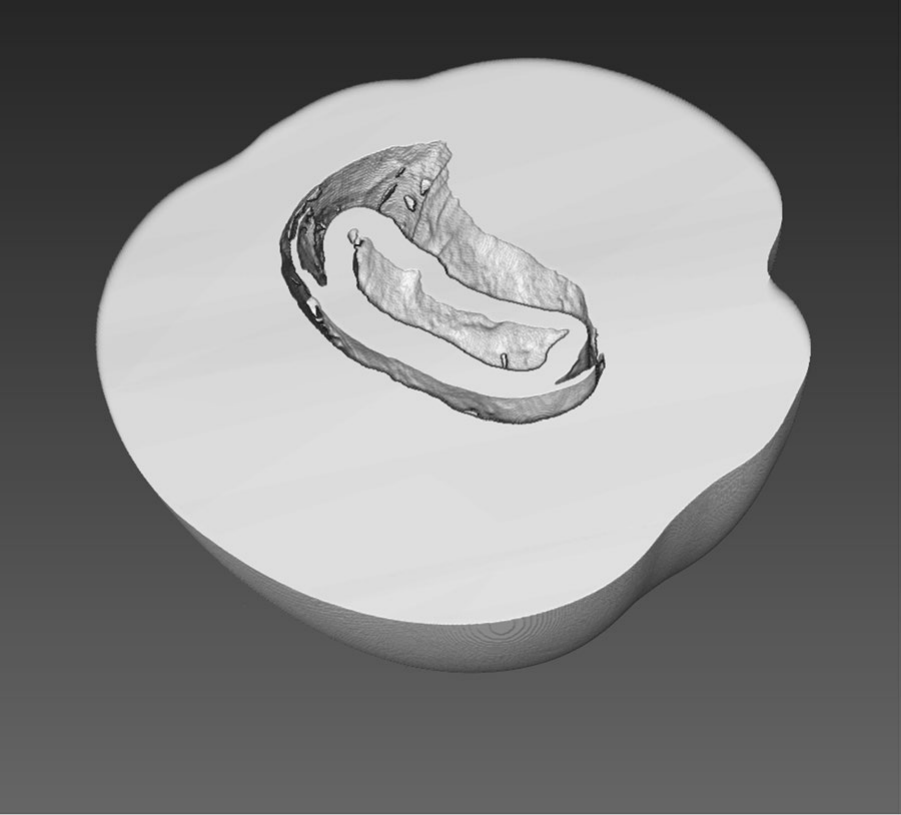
Volume rendering of the aragonite structure of the natural pearl NP-22 obtained from X-ray PC data.
